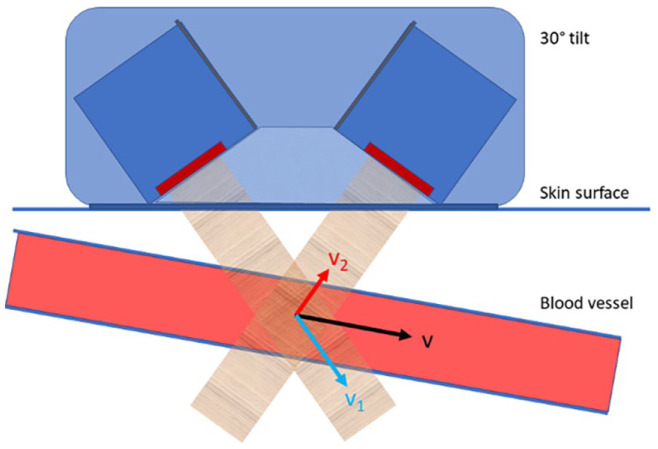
The earlybird dual-probe system consisted of two high-sensitive pulsed Doppler ultrasound probes. These probes were tilted 30° giving a fixed insonation angle of 60° to the skin surface, in a fixed 3D printed case, ensuring a consistent and known angle between them. A vector model-based algorithm calculated the peak systolic velocity (v) and diameter based on the respective velocities measured by the two probes (v1 and v2) and used to determine volume flow rate.
